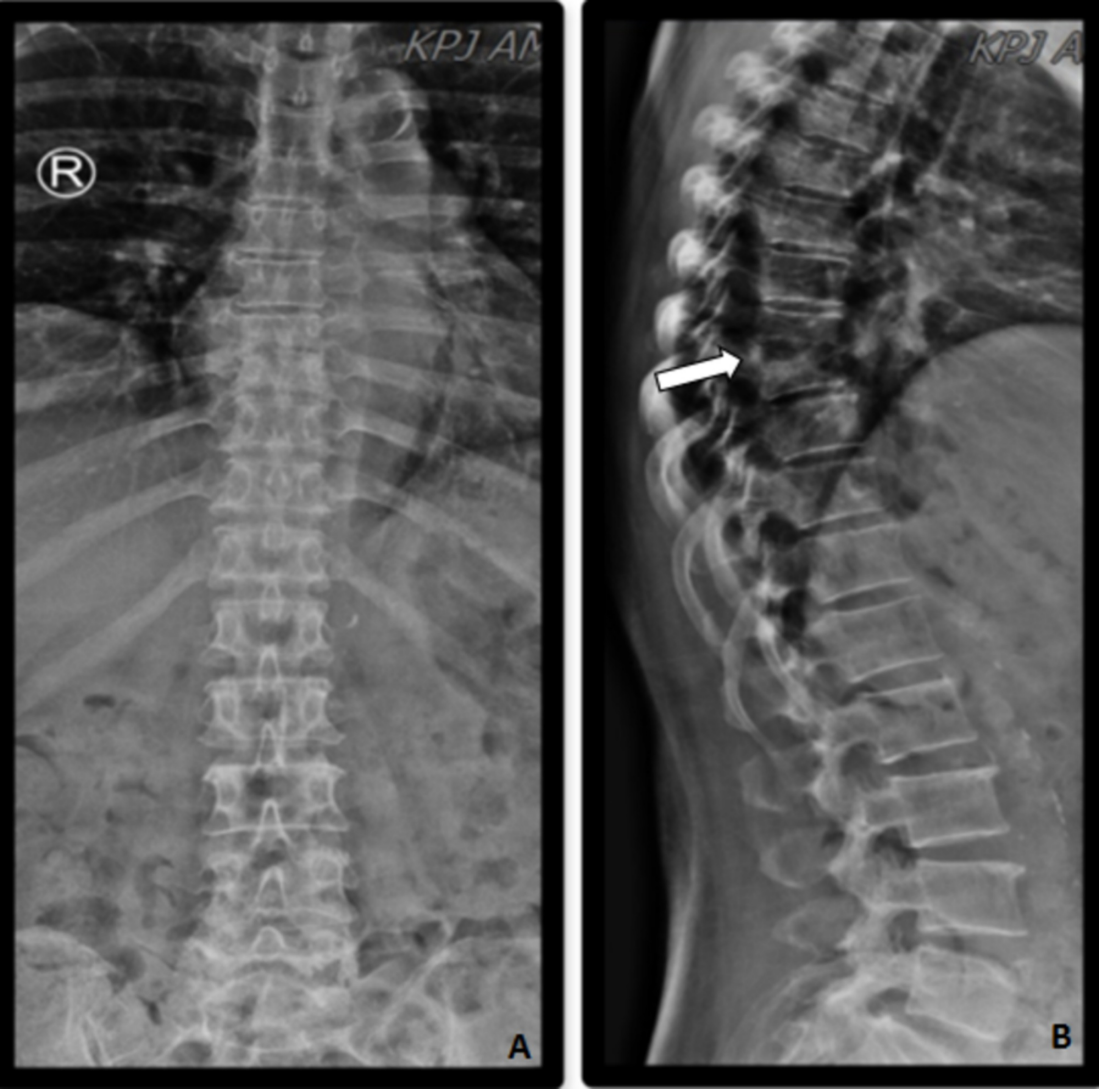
X-ray film of the spine at the two months follow-up. There is reduction of T8 and T9 vertebral body height, erosion of the superior endplate of T9 noted as shown in Figure 2B. The T8/T9 intervertebral disc space is narrowed. Appearance suggestive of discitis with paravertebral soft tissue collection at T8/T9 level and erosion of the T8 and T9 vertebra. A : antero-posterior view; B : lateral view
